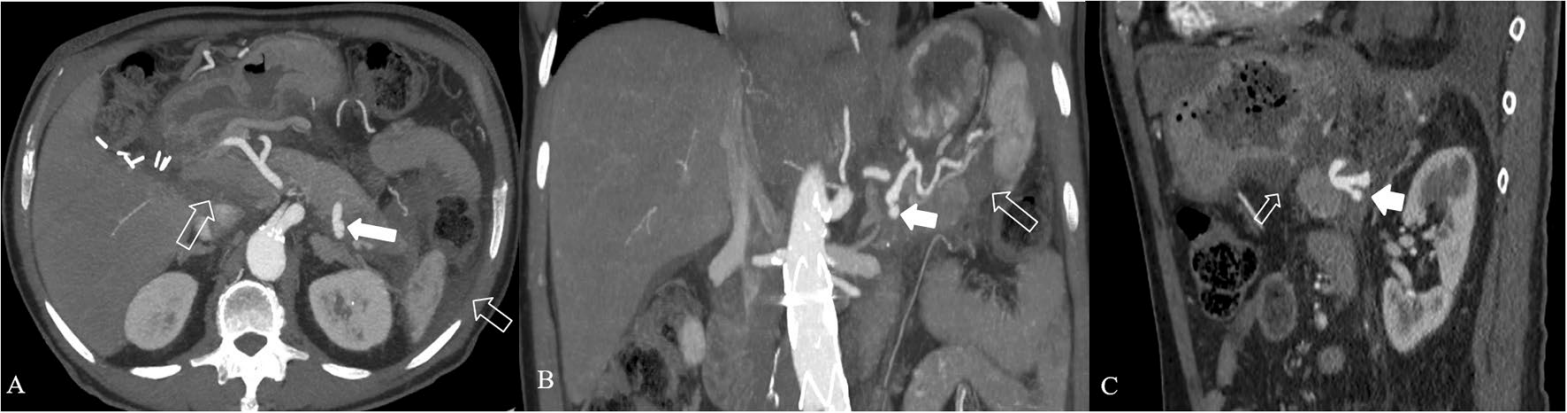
Splenic artery pseudoaneurism in a 75 years-old man with RAP. The contrast-enhanced CT scans in the arterial phase axial MIP (a), coronal MIP MPR (b) and sagittal MPR (c) show splenic artery pseudoaneurism (white arrows), in a necrotizing pancreatitis with evident peri-pancreatic collection (empty arrows) extending up to anterior pararenal space of the retroperitoneum, gastrohepatic and gastrosplenic
